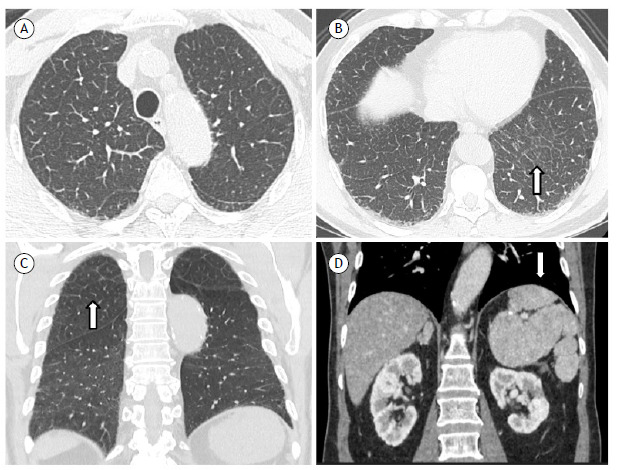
Chest CT shows diffuse septal thickening (arrows) and mild areas of emphysema predominantly in the upper lung lobes in A and B (axial reconstruction) and C (coronal reconstruction). In D, coronal reconstruction shows the remaining spleen (arrow).
